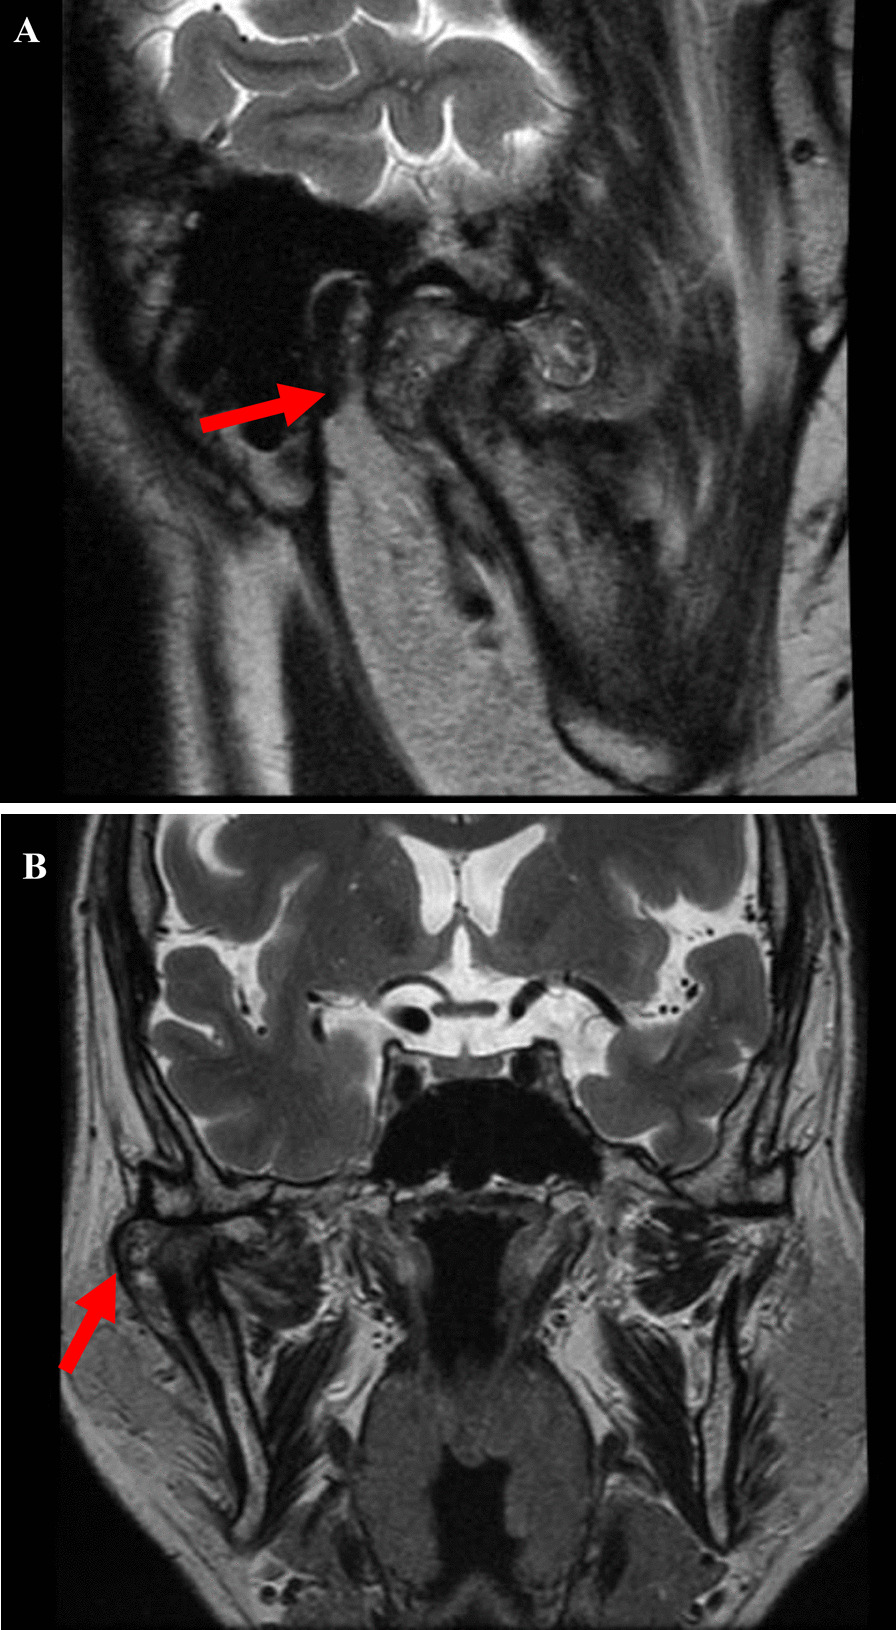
Preoperative MRI images. A Sagittal T1WI of the Right TMJ shows a fluid-filled mass expanding the joint space and multiple loose bodies. B Coronal PDWI showing heterogeneous signal intensity tissue mass within the capsule of the right TMJ between the right glenoid fossa and the mandibular condyle (red arrow)
